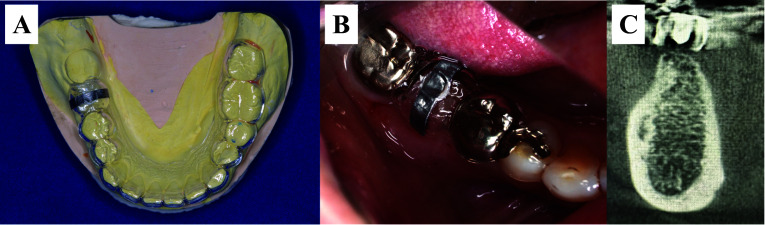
An example of radiopaque marker. A Radiopaque marker attached to the dental cast, with a circumferential lead foil around the crown and a vertical marker positioned perpendicular to the occlusal plane. B Intraoral placement of the radiopaque marker. C CT image showing the radiopaque marker clearly visible around the crown, with the vertical component facilitating three‑dimensional image alignment
